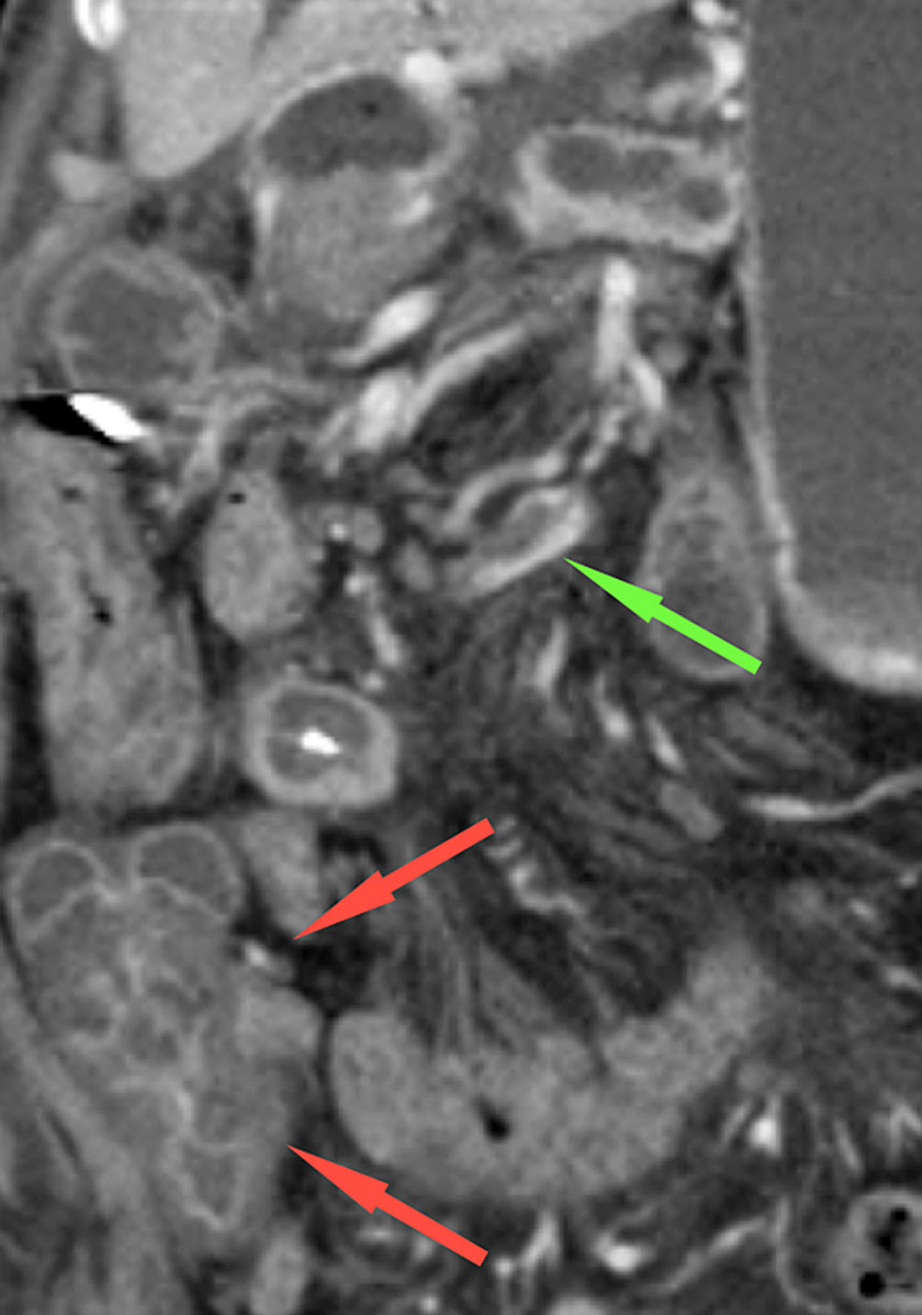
CT-image in the coronal plane, 1-week post-RFA, of a 68-year-old woman with a ductal adenocarcinoma located in the head and neck of the pancreas, showing a thrombus in the ileocolic vein (green arrow), resulting in edema of the ascending colon (red arrows)
